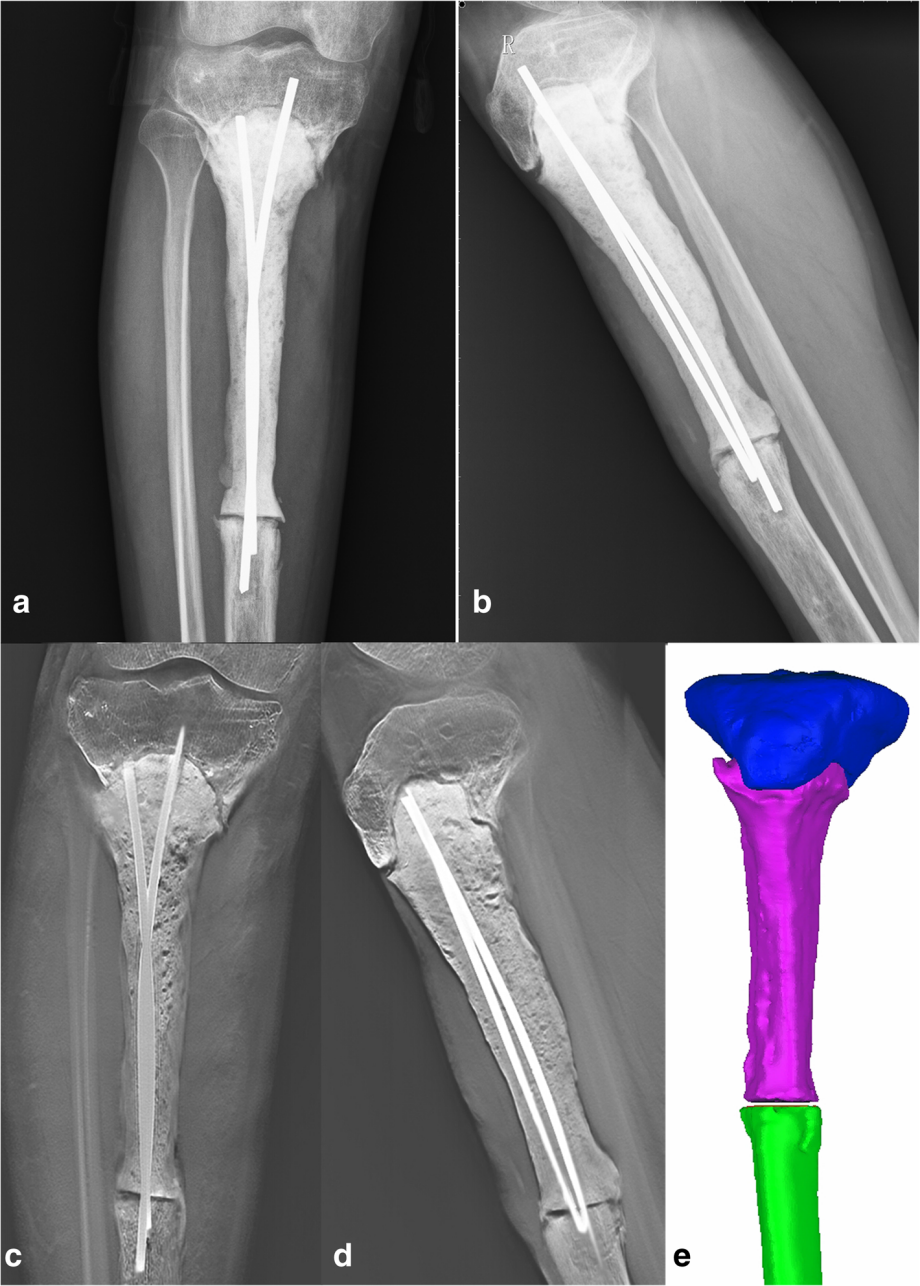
Anteroposterior (a) and lateral (b) radiographs of right tibia 9 months after temporary cemented spacer reconstruction. A distinct gap between the proximal tibia and the cemented spacer could be observed in anteroposterior (c) and lateral (d) T-SMART scanning. e Reconstructed 3D models of the proximal tibia, cemented spacer, and distal tibia
